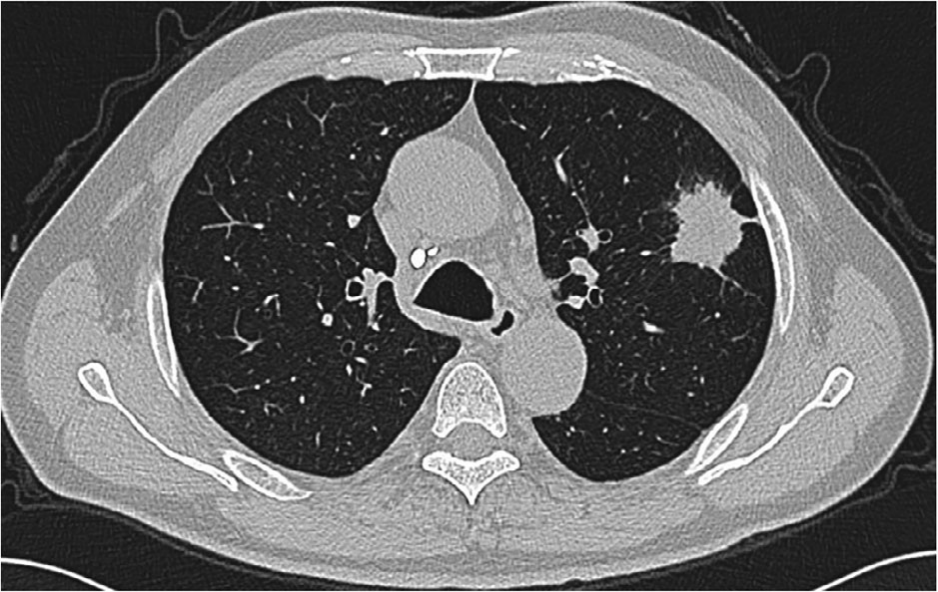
Chest computed tomography (CT) scan revealed a 3.8 × 3.4 cm mass in the left upper lobe of patient No. 4.
